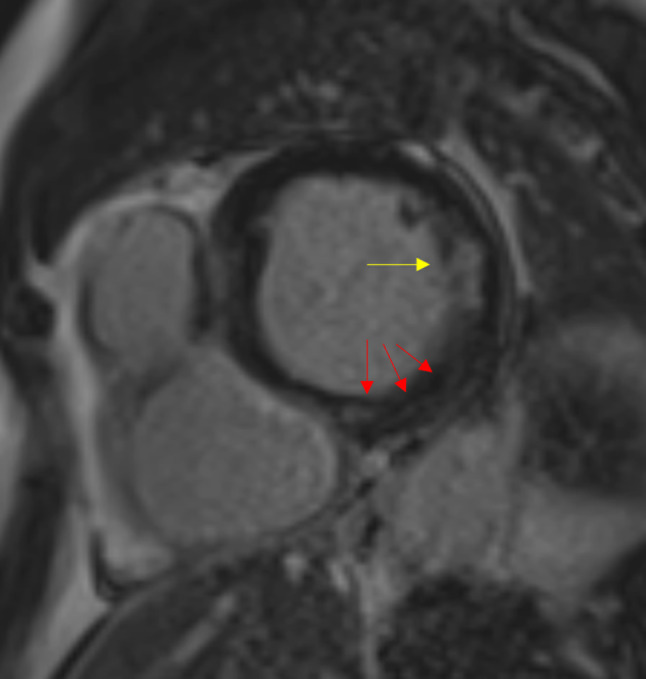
Cardiac magnetic resonance image showing subendocardial lateral myocardial infarction (*yellow arrow*) and foci of myocardial inflammation (*red arrows*)
